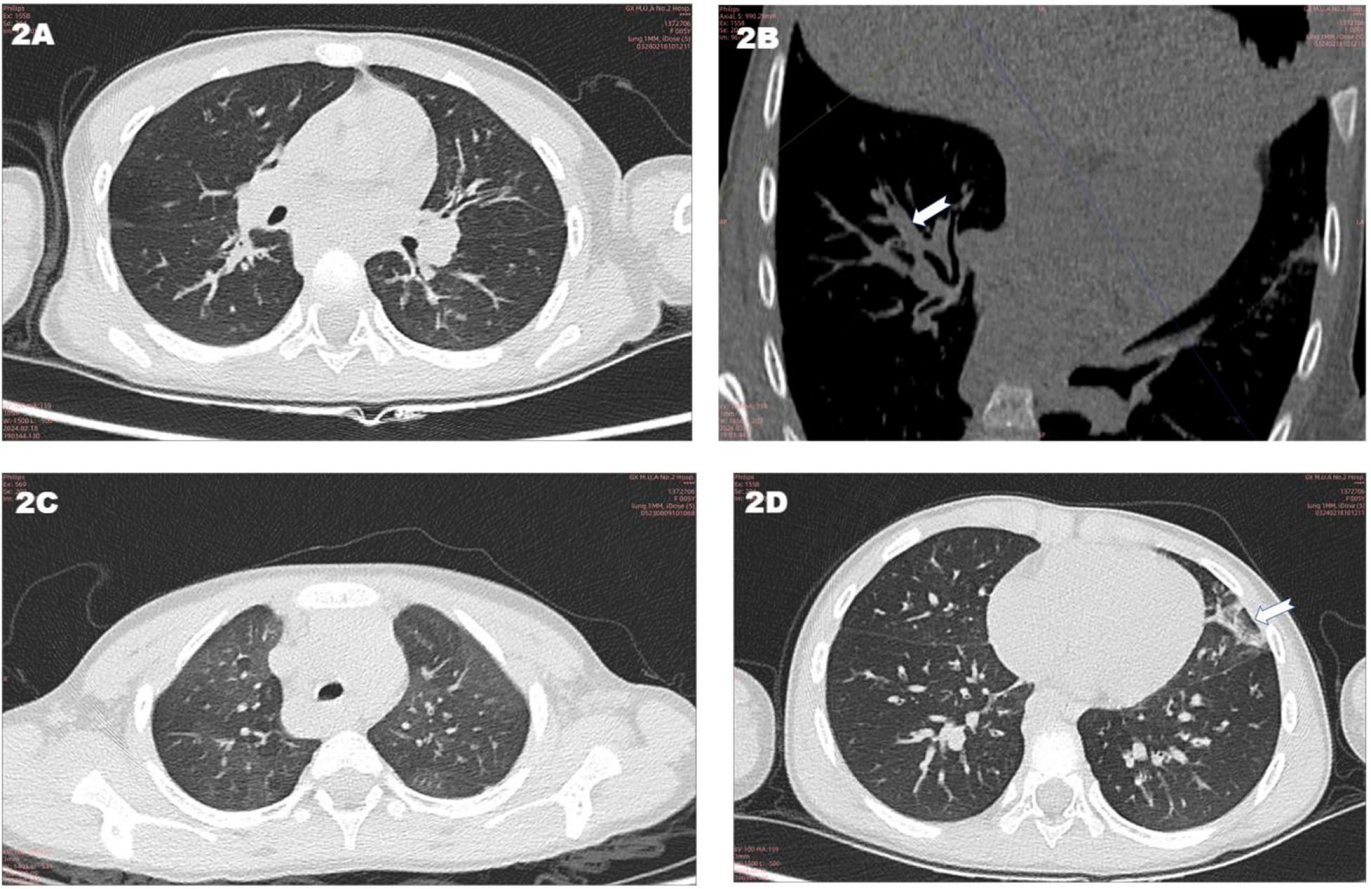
Chest computed tomography (CT) findings: A axial CT image of the chest (lung window) shows a well-defined nodular lesion in the left hilum, with no obvious bronchial obstruction, B coronal reconstructed CT image (mediastinal window) reveals focal stenosis and irregularity of the right middle lobe bronchus (arrow), suggesting bronchial involvement, C axial CT image of the upper chest (lung window) demonstrates multiple small nodular opacities bilaterally, predominantly in the peripheral lung zones, D axial CT image of the lower chest (lung window) shows patchy ground-glass opacities and small nodules in the left lower lung, with a focal area of consolidation (arrow).
